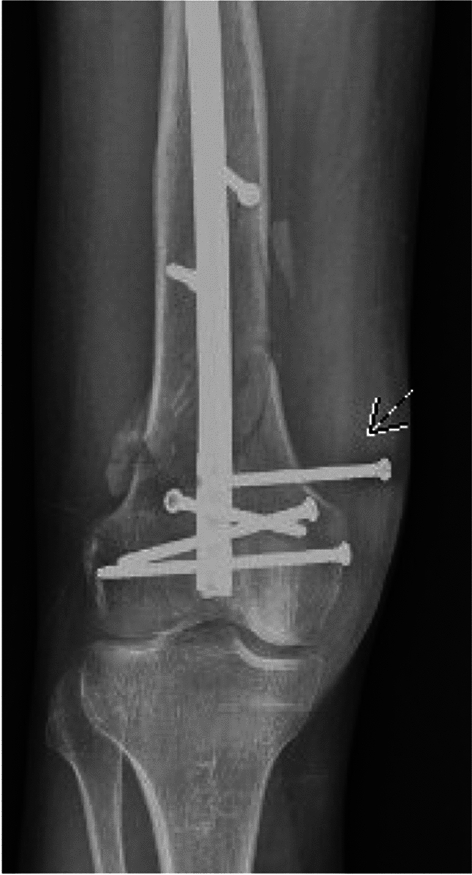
Follow-up AP radiograph demonstrating 27.7 mm distal interlock screw back-out four weeks after treatment with RFNA. The alignment remained unchanged
